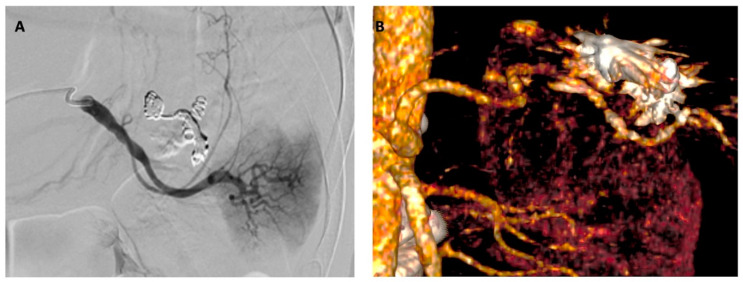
(A) Completion angiography showing complete splenic artery exclusion after coil embolization, and (B) postoperative three-dimensional volume rendering CTA reconstruction confirming the complete aneurysm exclusion.
